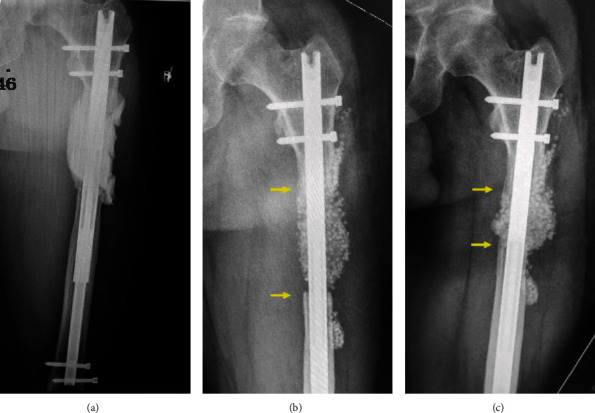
First procedure. Radiographs of the left femur showing the cement spacer (a), the graft aspect at five months after the first grafting (b), and the graft collapse following nail dynamization (c).
